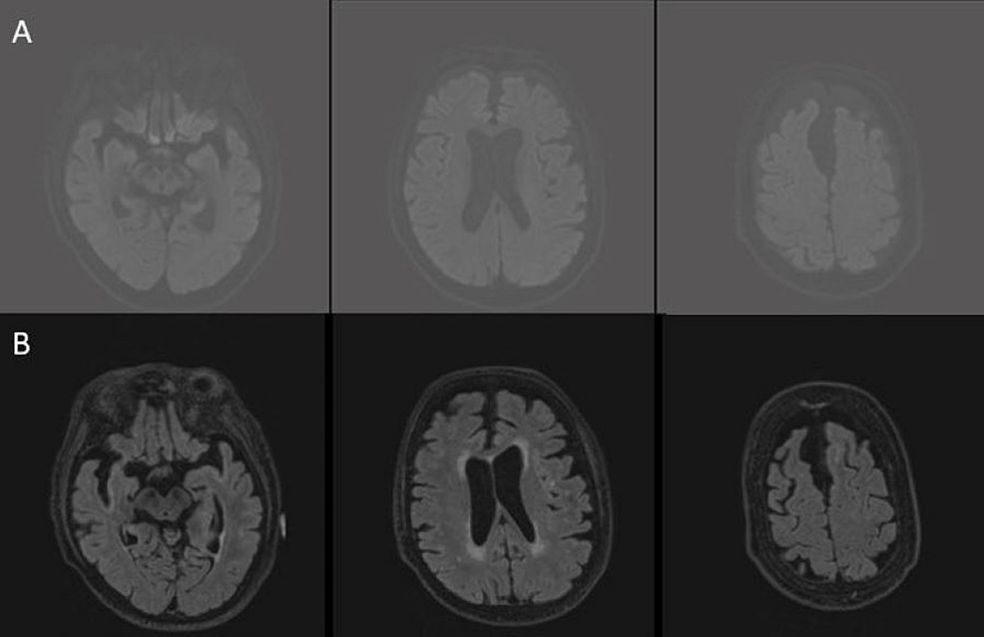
Follow-up MRI shows regression of the cortical lesions previously demonstrated both on DW (A) and FLAIR images (B). FLAIR: fluid‐attenuated inversion recovery; DW: diffusion-weighted
